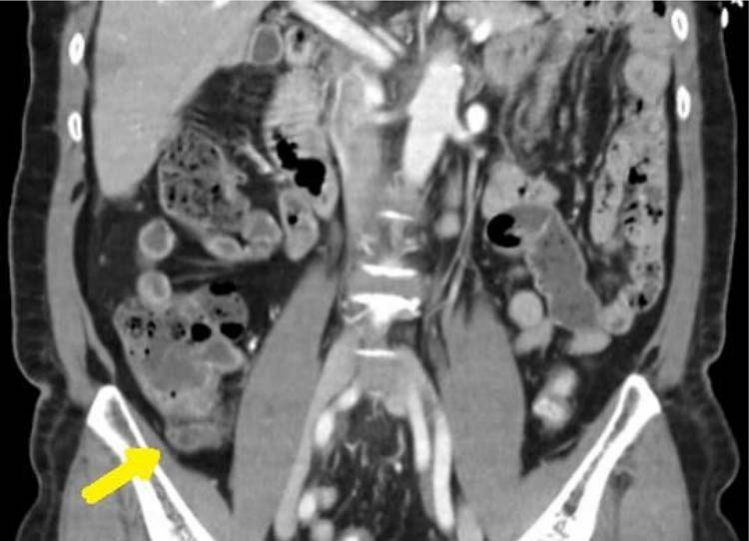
CT scan coronal view: dilated appendix with fat stranding.
